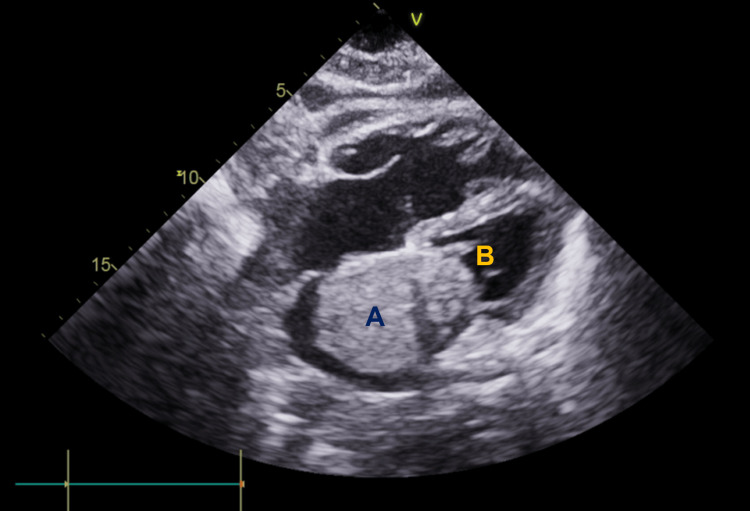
Transthoracic echocardiogram with a clear intracardiac mass (A) obstructing the mitral valve (B) in diastole.
